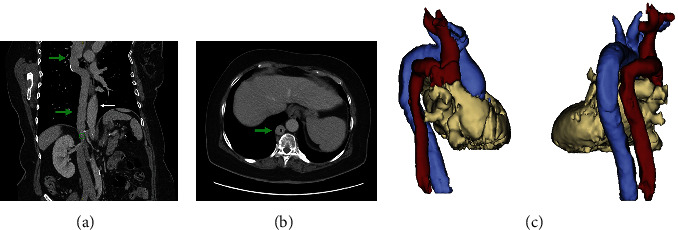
(a) Stretched reconstruction of a venous CT with azygos continuation (green arrows), aorta (white arrow), and diaphragmatic passage of the azygos (green circle). (b) Axial CT view, venous phase, showing a prominent azygos vein (green arrow). (c) 3D reconstruction showing the azygos continuation/superior vena cava (red) and the aortic arch (blue) in a right lateral and posterior view.
